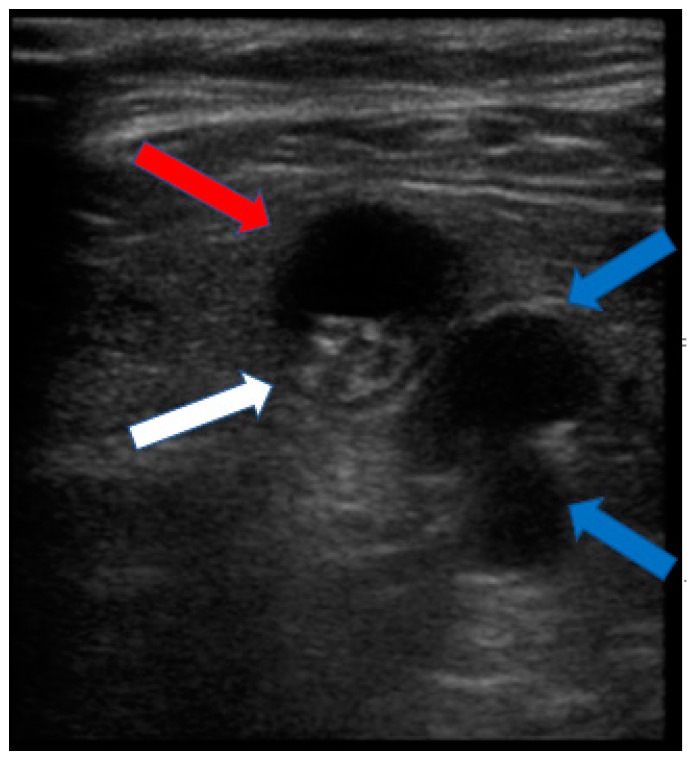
Ultrasound showing several cysts compressing the peroneal nerve around the distal femur level (red arrow: ganglion cyst; white arrow: compressed peroneal nerve; blue arrow: popliteal artery and vein).
